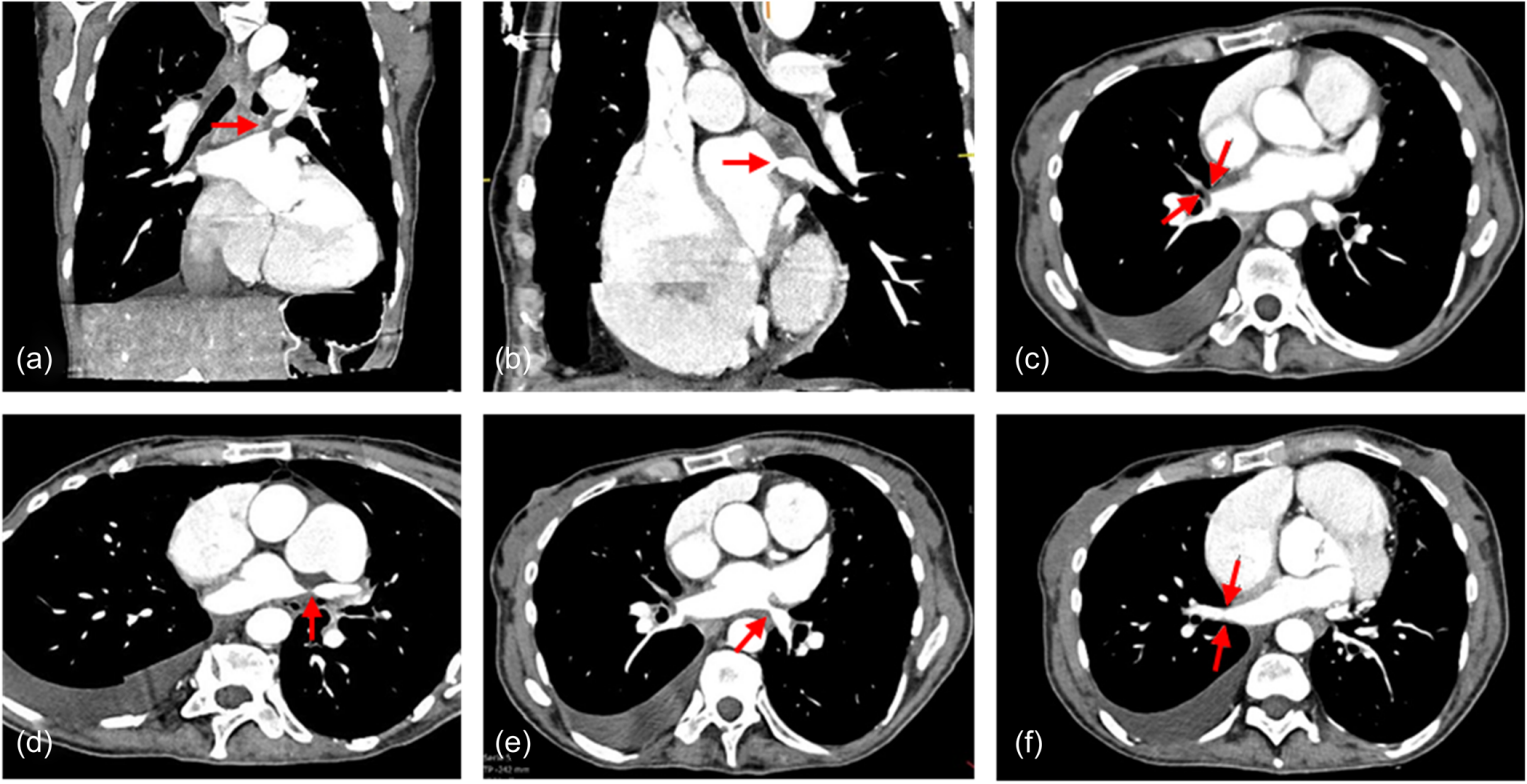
CT angiography at diagnosis of pulmonary vein stenosis. (a, d) Total occlusion of LSPV. (b, e) Stenosis of LIPV. (c) Total occlusion of RSPV in segment 3. (f) Stenosis of RIPV. CT, computed tomography; LIPV, left inferior pulmonary vein; LSPV, left superior pulmonary vein; RIPV, right inferior pulmonary vein; RSPV, right superior pulmonary vein.
